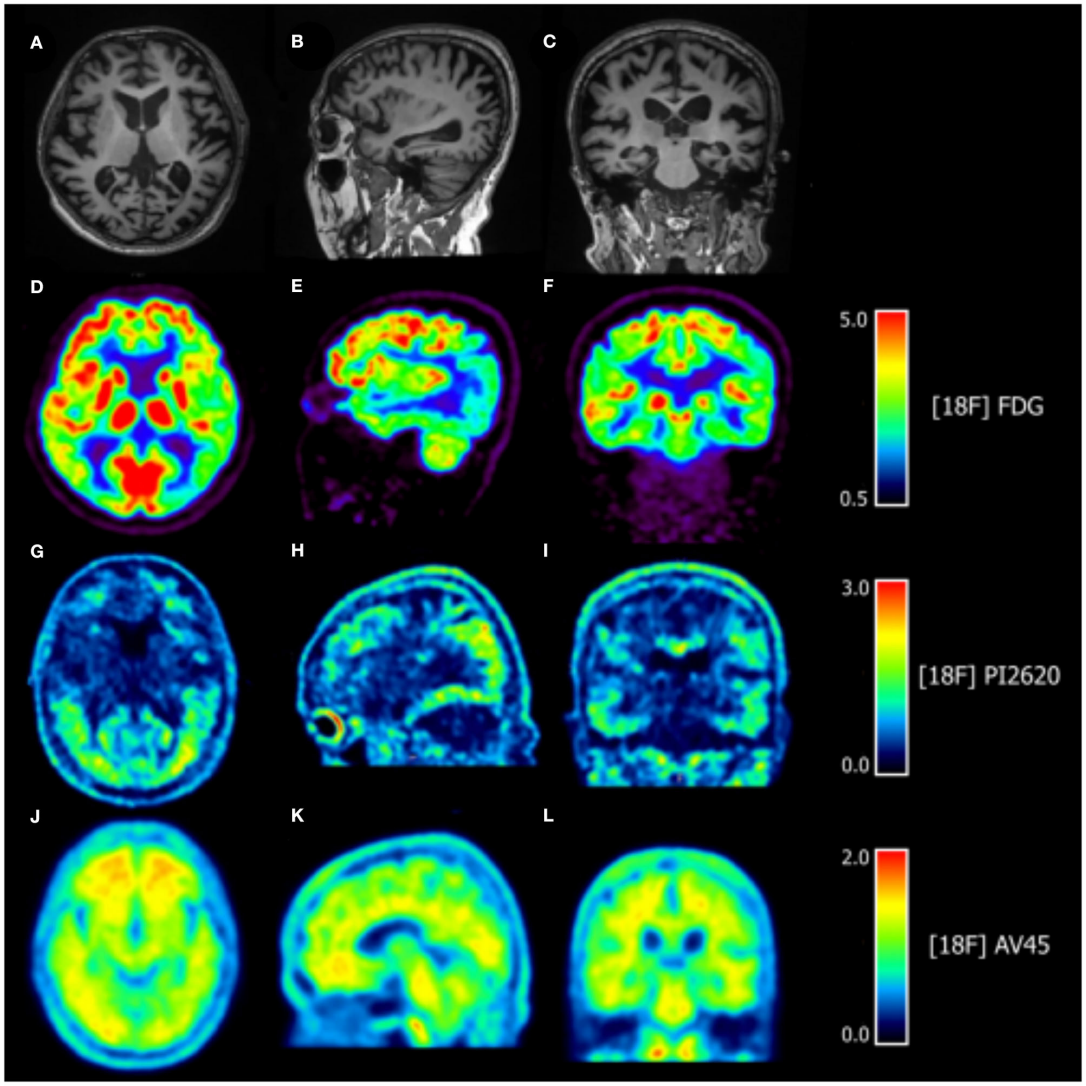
Axial, sagittal, and coronal slices from MRI and [^18^F] fluorodeoxyglucose ([^18^F]FDG), [^18^F]PI2620, and [^18^F]AV45 PET scans. (A–C) T1-weighted sequences in MRI show cortical and subcortical atrophy, especially in bilateral parietal and occipital lobes. (D–F) [^18^F]FDG PET revealed significant hypometabolism in diffuse regions of bilateral temporal, parietal, and occipital lobes, prominently in occipital lobes, and no metabolic change was observed in the frontal lobes, basal ganglia, and thalami. (G–I) [^18^F]PI2620 PET showed increased tracer uptake throughout the brain, especially in the temporal, parietal, and occipital lobes. (J–L) [^18^F]AV45 PET showed amyloid deposition throughout the cortex that was especially pronounced in bilateral frontal cortices.
